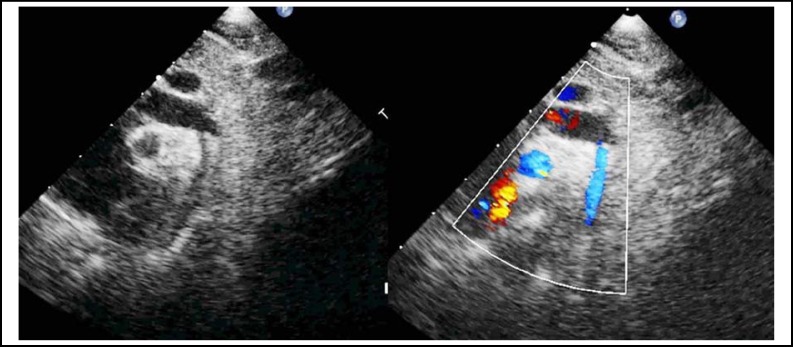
A two-dimensional and color Doppler echocardiographic image obtained from 14 days neonate
